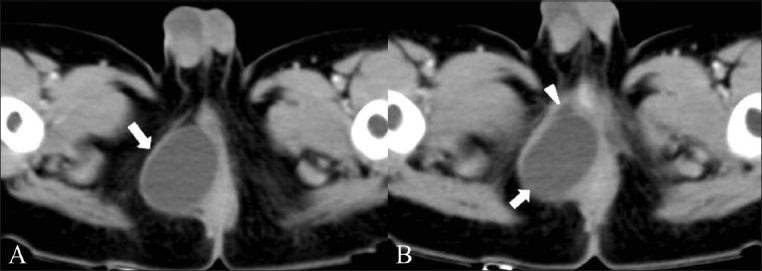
Axial CT scans show the tailgut cyst as a round, sharply-marginated lesion (arrow) with fluid contents, displacing the anal canal to the left, with a smaller peripheral cyst (arrowhead in B) seen anterior and inferior to the larger cyst
